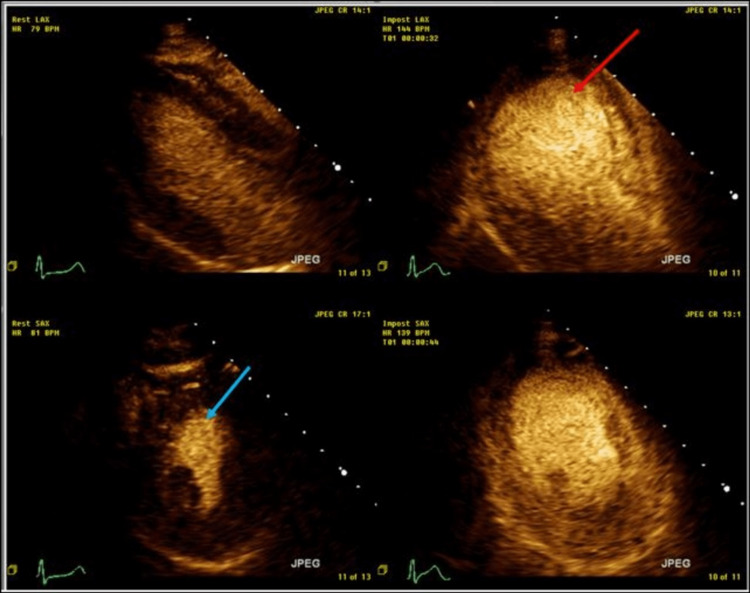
Echocardiographic images of the left ventricle at rest and during stress. Echocardiographic images of the left ventricle demonstrate adequate thickening and contraction of the myocardium at rest (blue arrow) as compared to exercise-induced global dilatation and apical ballooning of the left ventricle at stress (red arrow).
